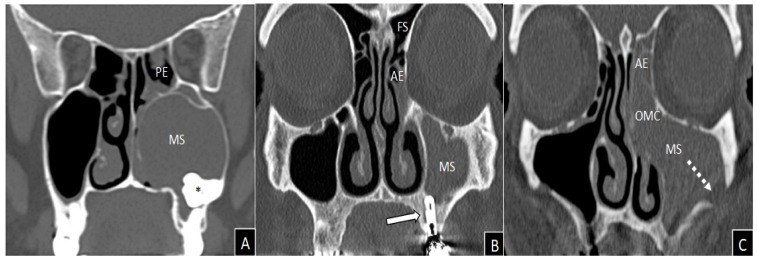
Coronal views of computed tomography imaging. Massive and homogeneous left maxillary sinus opacification in a non-eosinophilic polypoid oCRS (tissue eosinophils count 0/5HPF) caused by an included tooth (asterisk), which deforms the medial wall of the maxillary sinus causing obliteration of the ostiomeatal complex (A). Left anterior ethmoidal and maxillary sinuses inflammatory involvement in a patient with non-eosinophilic oCRS without polyps (tissue eosinophils count 10/5HPF) caused by dental implantation of element 2.6 (white arrow) (B). Massive inflammation of left maxillary and anterior ethmoid with obliteration of the ostiomeatal complex in a patient with oro-antral fistula (white dashed arrow) and non-polypoid eosinophilic oCRS (tissue eosinophils count 22/5HPF) caused by zygomatic implant (C). AE = anterior ethmoid; MS = maxillary sinus; OMC = ostiomeatal complex; PE = posterior ethmoid; FS = frontal sinus.
